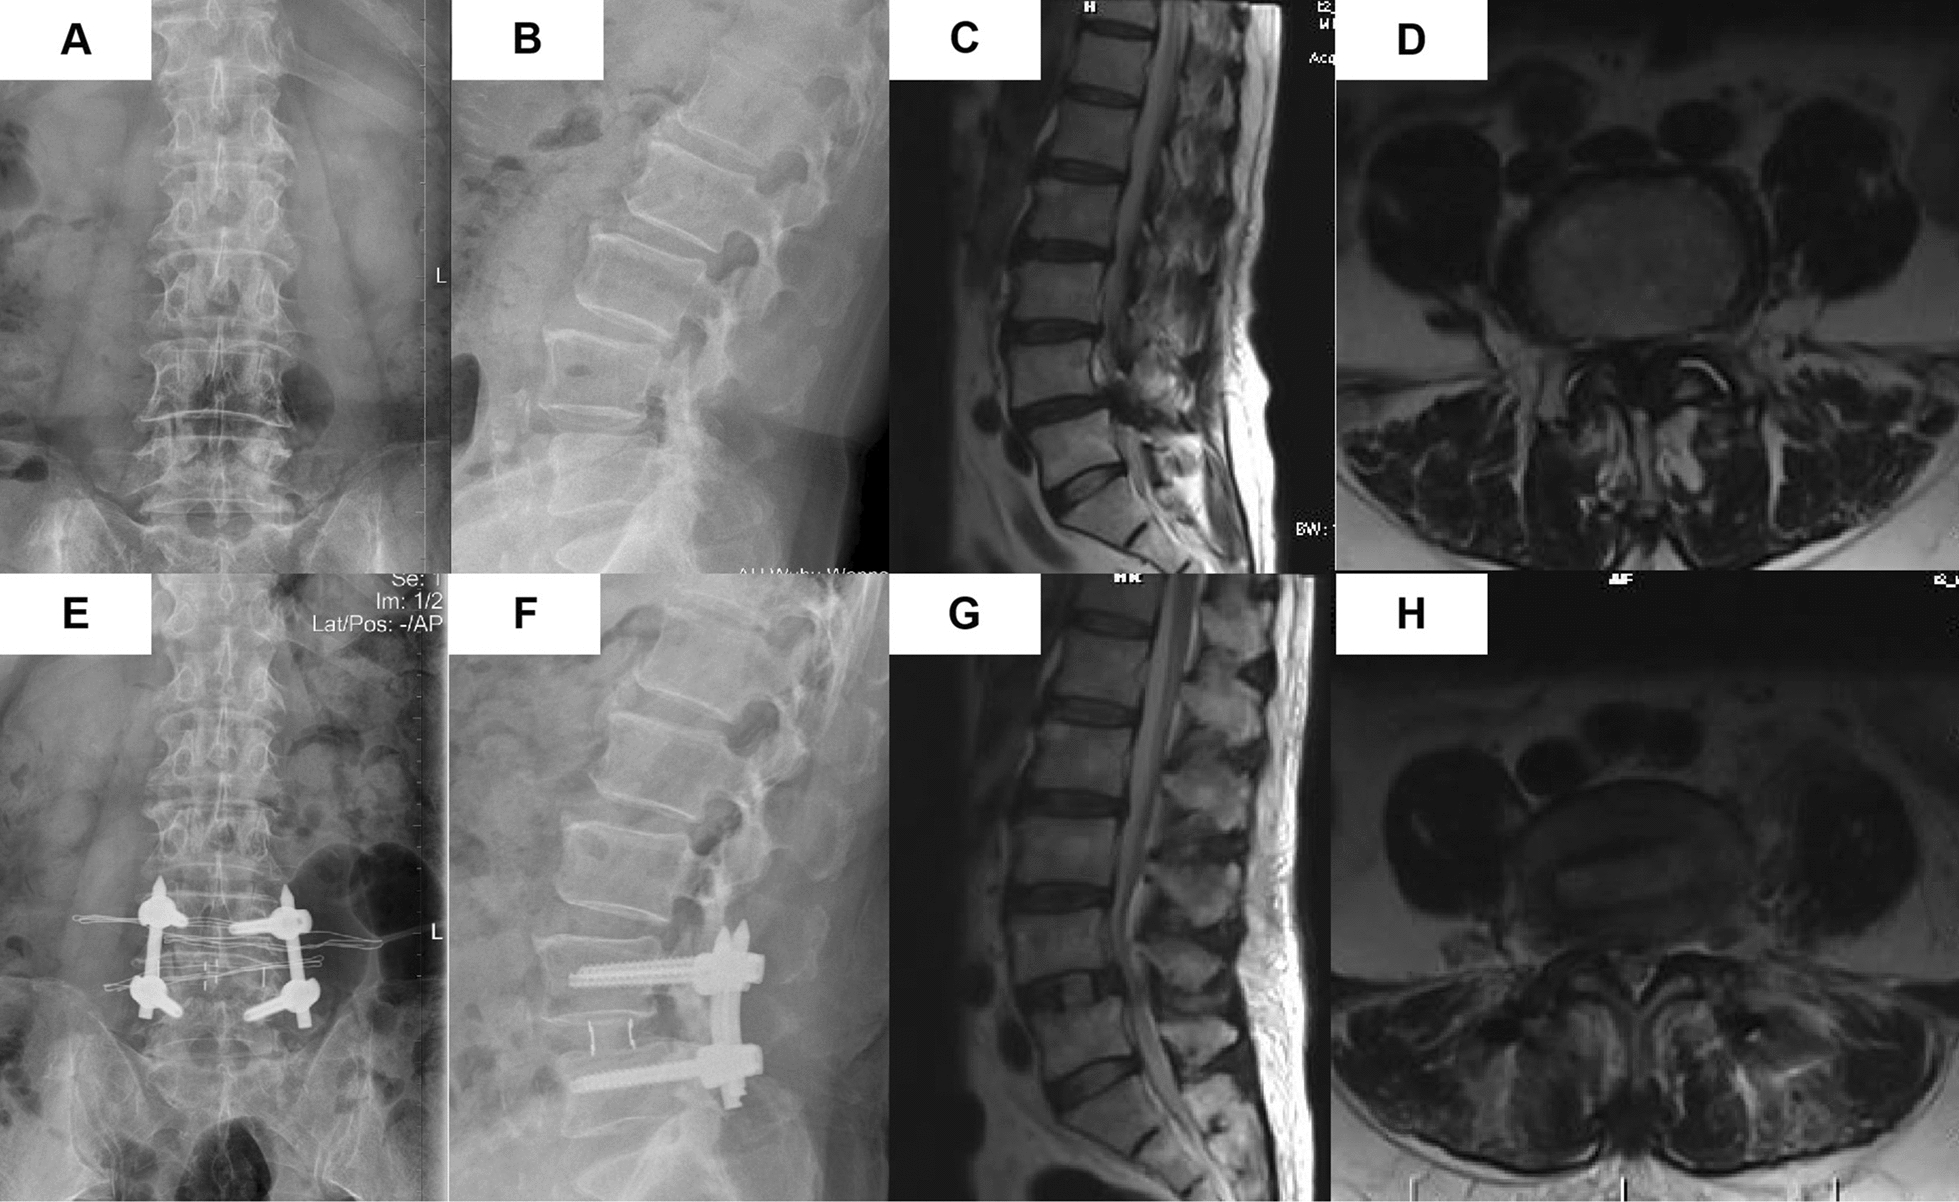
A typical case of OLIF. A, B The preoperative anteroposterior and lateral radiographs demonstrate Grade 1 spondylolisthesis at L4/5. E, F The preoperative sagittal and coronal MRI shows severe central stenosis (Schizas Grade D) at L4/5. C, D The postoperative anteroposterior and lateral radiographs one day after OLIF. G, H The preoperative sagittal and coronal MRI showed (Schizas Grade B) at L4/5
